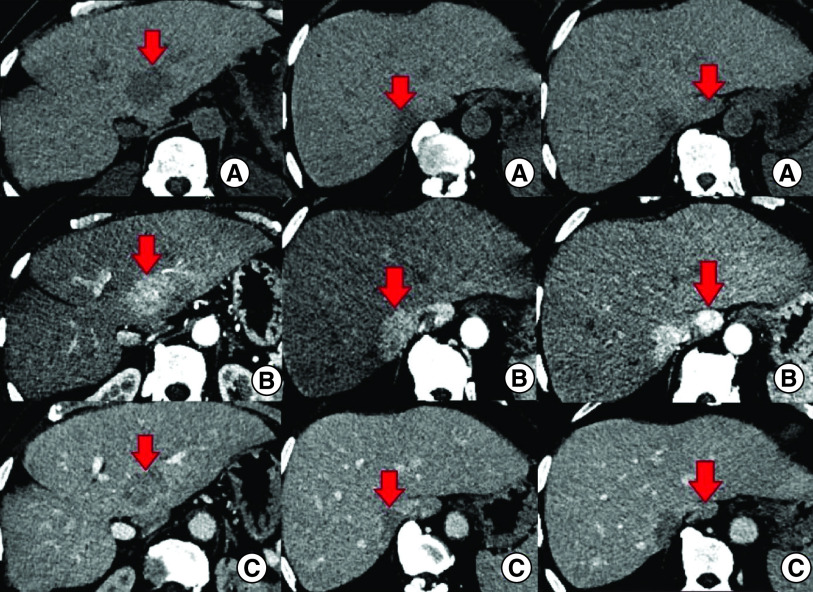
Abdominal CT – axial sections showing the hepatic lesions (red arrows). (A) The lesions are spontaneously hypodense. (B) After injection of contrast product, the lesions are intensely enhancing in the arterial phase. (C) Contrast product wash out in the portal phase.
